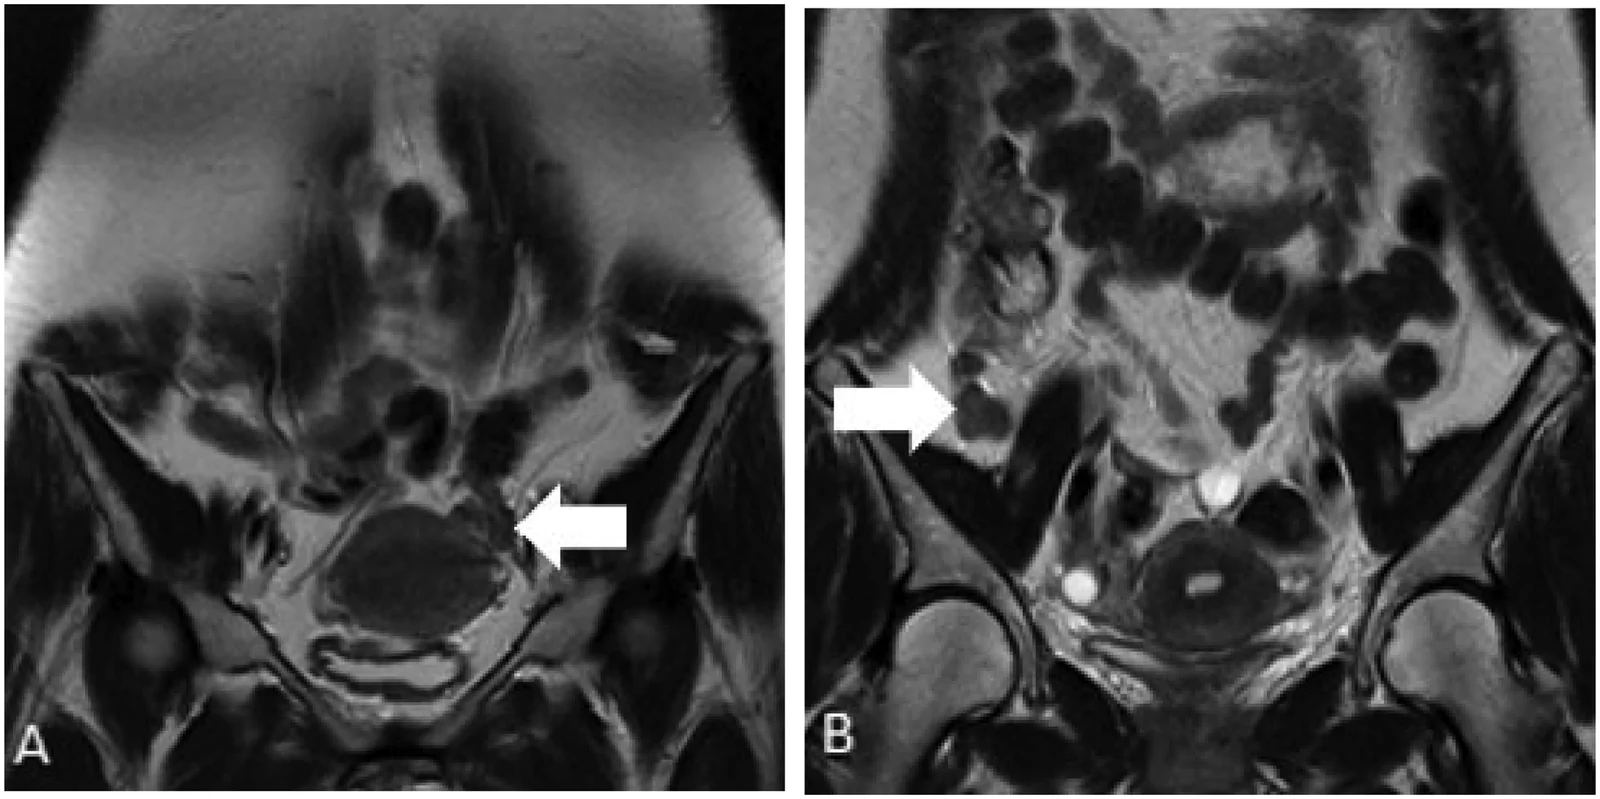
MRI of the abdomen and pelvis in coronal T2WI showing a similar signal pattern to that of the solid pedunculated uterine/operative bed leiomyoma (A) and a nodular peritoneal lesion adjacent to the right distal ileal loop (B).
